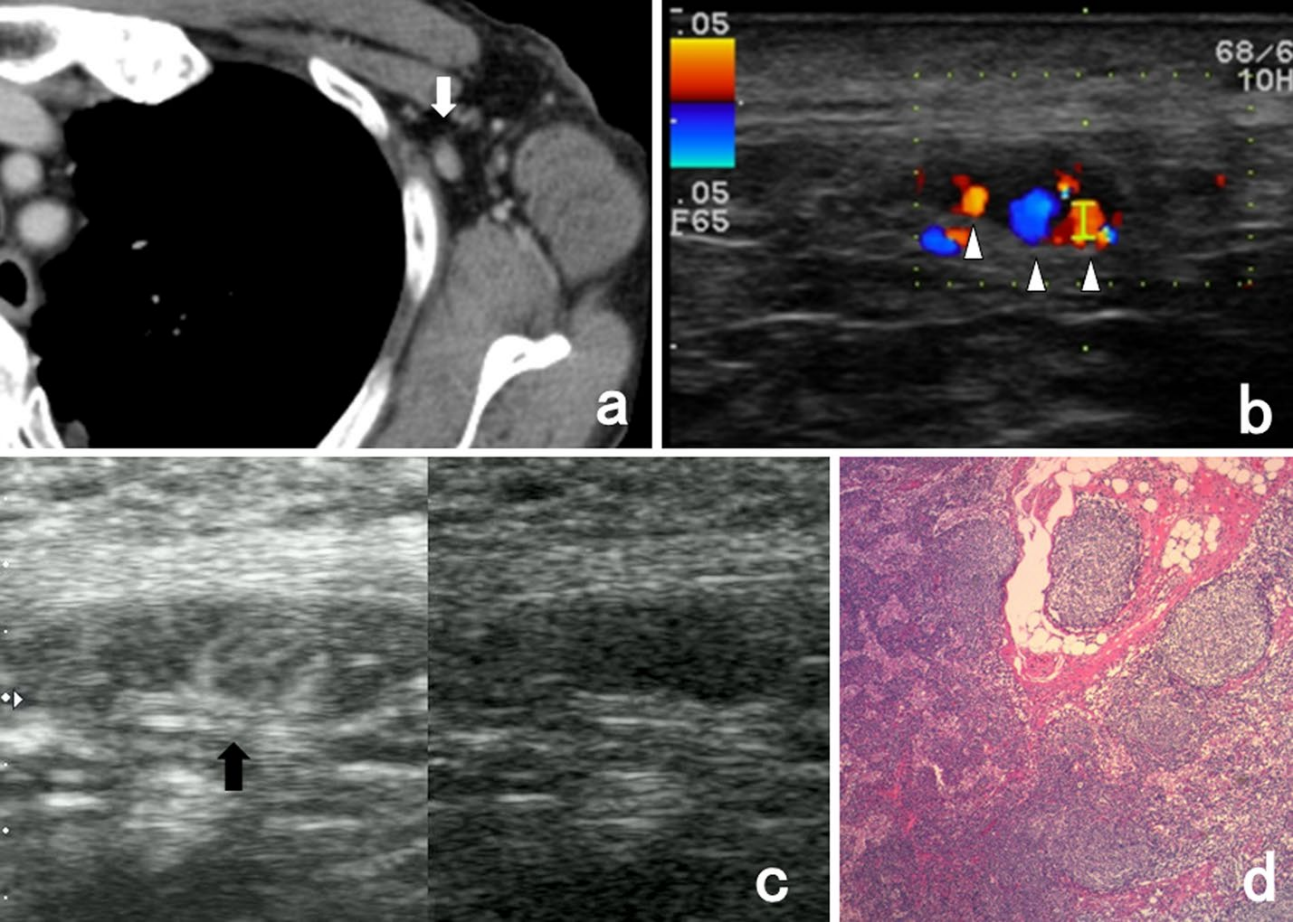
Representative images of a patient with pathologically negative metastasis of axillary lymph node. CECT images showed moderate-enhanced axillary lymph node (a). Color Doppler US showed blood flows from multiple micro vessel (b). CEUS imaging with Sonazoid revealed that these flows origin from a single vessel in the hilum (c). Pathological findings showed the negative metastasis of this axillary lymph node (d). *White arrow* shows the axillary sentinel lymph node. *White arrow heads* show the micro vessel from the other places of hilum. *Black arrow* shows the blood flows from a single micro vessel
